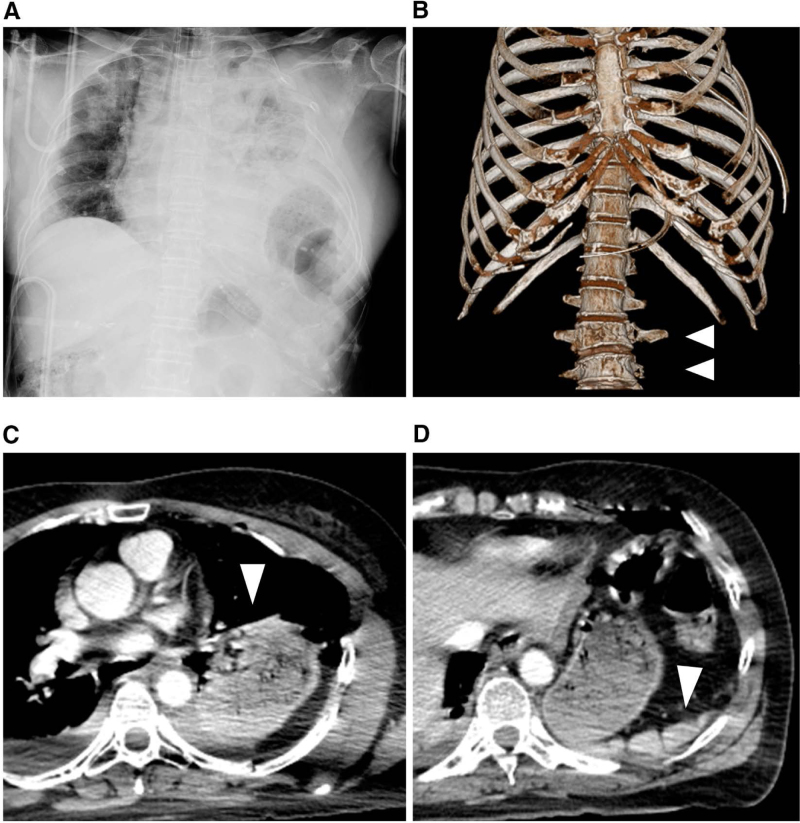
Radiographic imaging on admission. (A) Chest radiograph shows a left diaphragmatic injury with a hernia and multiple rib fractures. (B) Computed tomography (CT) shows the ruptured lumbar vertebral fractures (white arrowheads). (C, D) CT shows the left diaphragmatic injury and hernia and splenic injury (white arrowhead). The diaphragmatic injury corresponds to an AAST-OIS grade IV. AAST-OIS = American Association for the Surgery of Trauma–Organ Injury Scale, CT = computed tomography.
